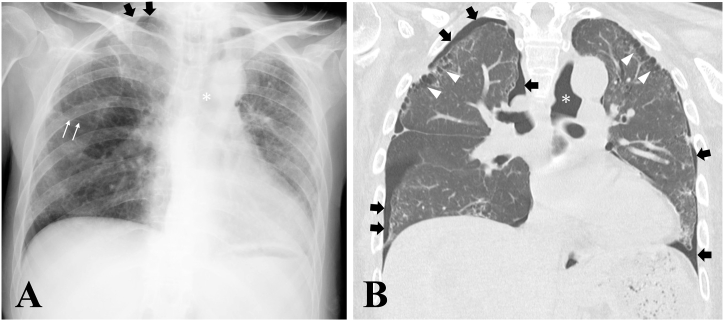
Chest radiograph and computed tomography findings (A) Chest radiograph showing bilateral upper lobe volume loss with hilar elevation and platythorax. (B) Coronal chest CT demonstrating bilateral upper-lobe-dominant subpleural fibrosis with honeycombing and traction bronchiectasis. Thin white arrows indicate elevated fissures due to upper lobe volume loss; thick black arrows indicate pneumothorax; asterisks indicate pneumomediastinum; white arrowheads indicate bilateral upper-lobe-dominant subpleural fibrosis with honeycombing.
